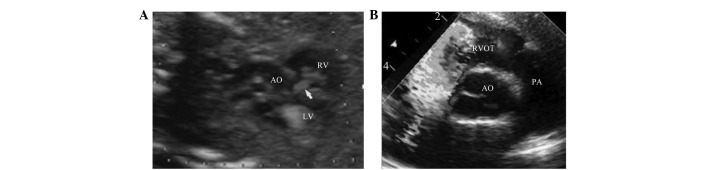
A 25-week fetus was diagnosed with a ventricular septal defect of the membranous part by echocardiography, but a echocardiography recheck in the 42-day infant indicated a normal heart. (A) Fetal echocardiography: color Doppler indicating left to right shunting signal of the left ventricle of the outflow tract (arrow). (B) Infant echocardiography: color Doppler indicating that no ventricular level shunting signal of the membranous ventricular septum was present in the aorta short axis view. AO, aorta; LV, left ventricle; RV, right ventricle; RVOT, right ventricle of outflow tract; PA, pulmonary artery.
